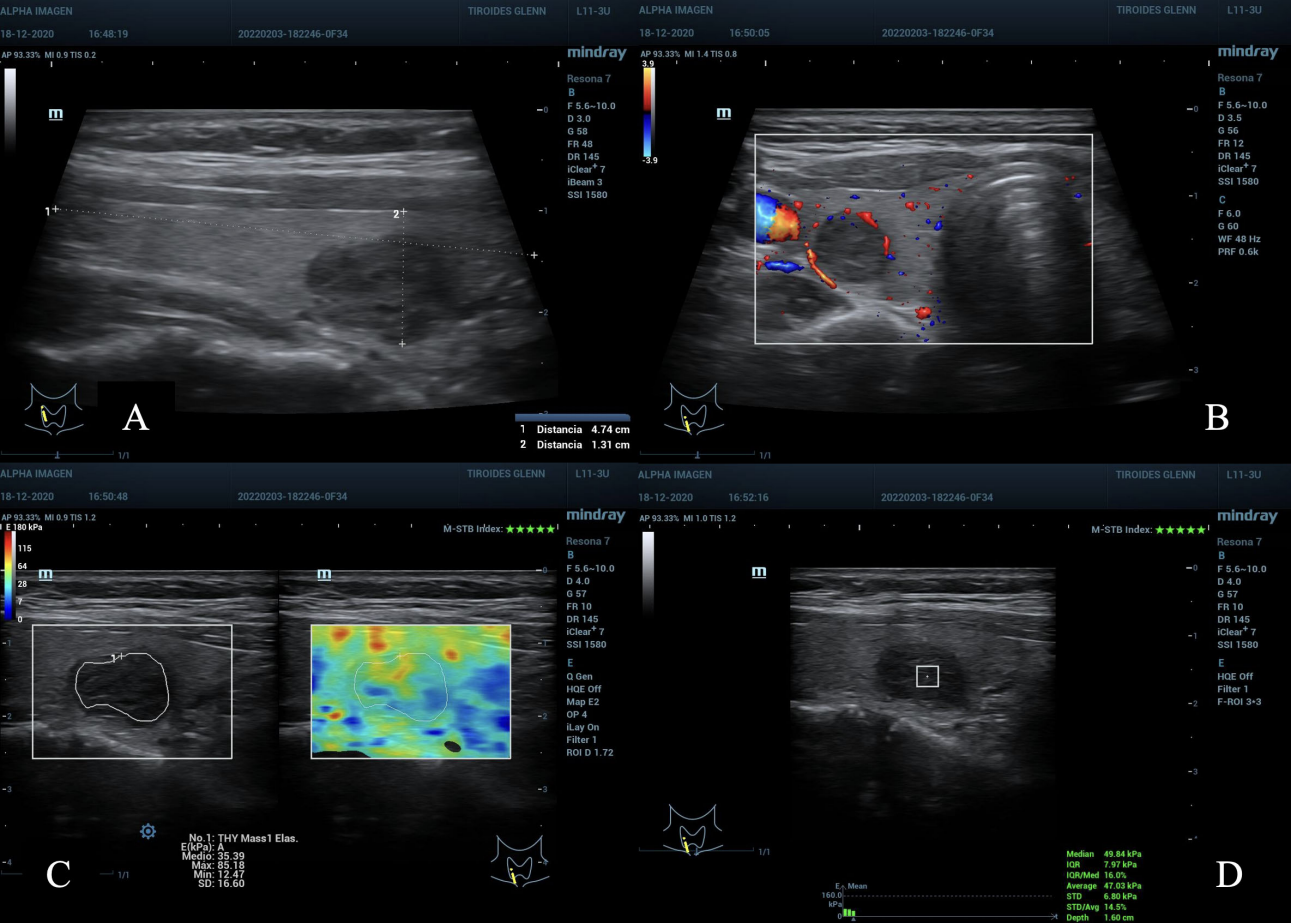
(A) nodule in the lower right third of the thyroid gland, maximum diameter 2.0 cm, ACR TI-RADS 4. (B) peripheral Doppler vascularization, AS moderate suspicion. (C) maximum scale of 180kPa, TE 2D-SWE Emean and Emax with kPa below C/O. (D) pSWE kPa below C/O, MIQR 16%, ROI box 3 x 3 mm, depth 1.6 cm. Result: cytopathological Bethesda: II, benign.
